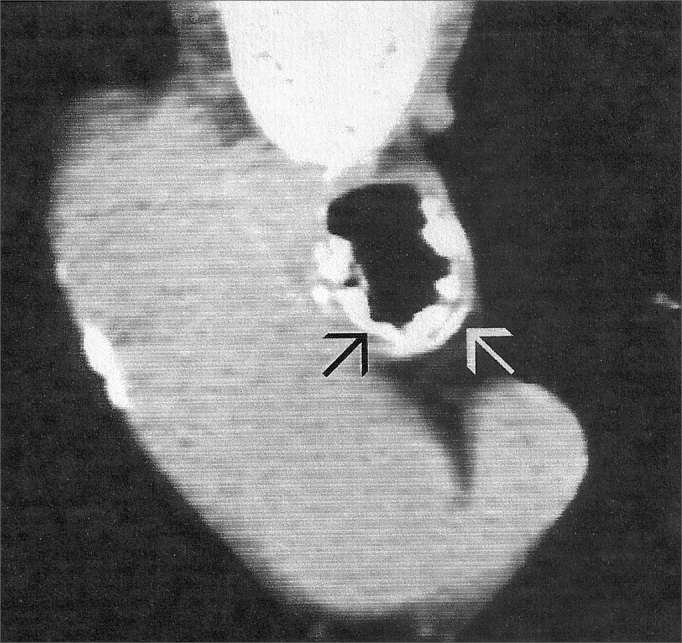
Tracheal CT scan showing osteocartilaginous nodular lesions (arrows).
